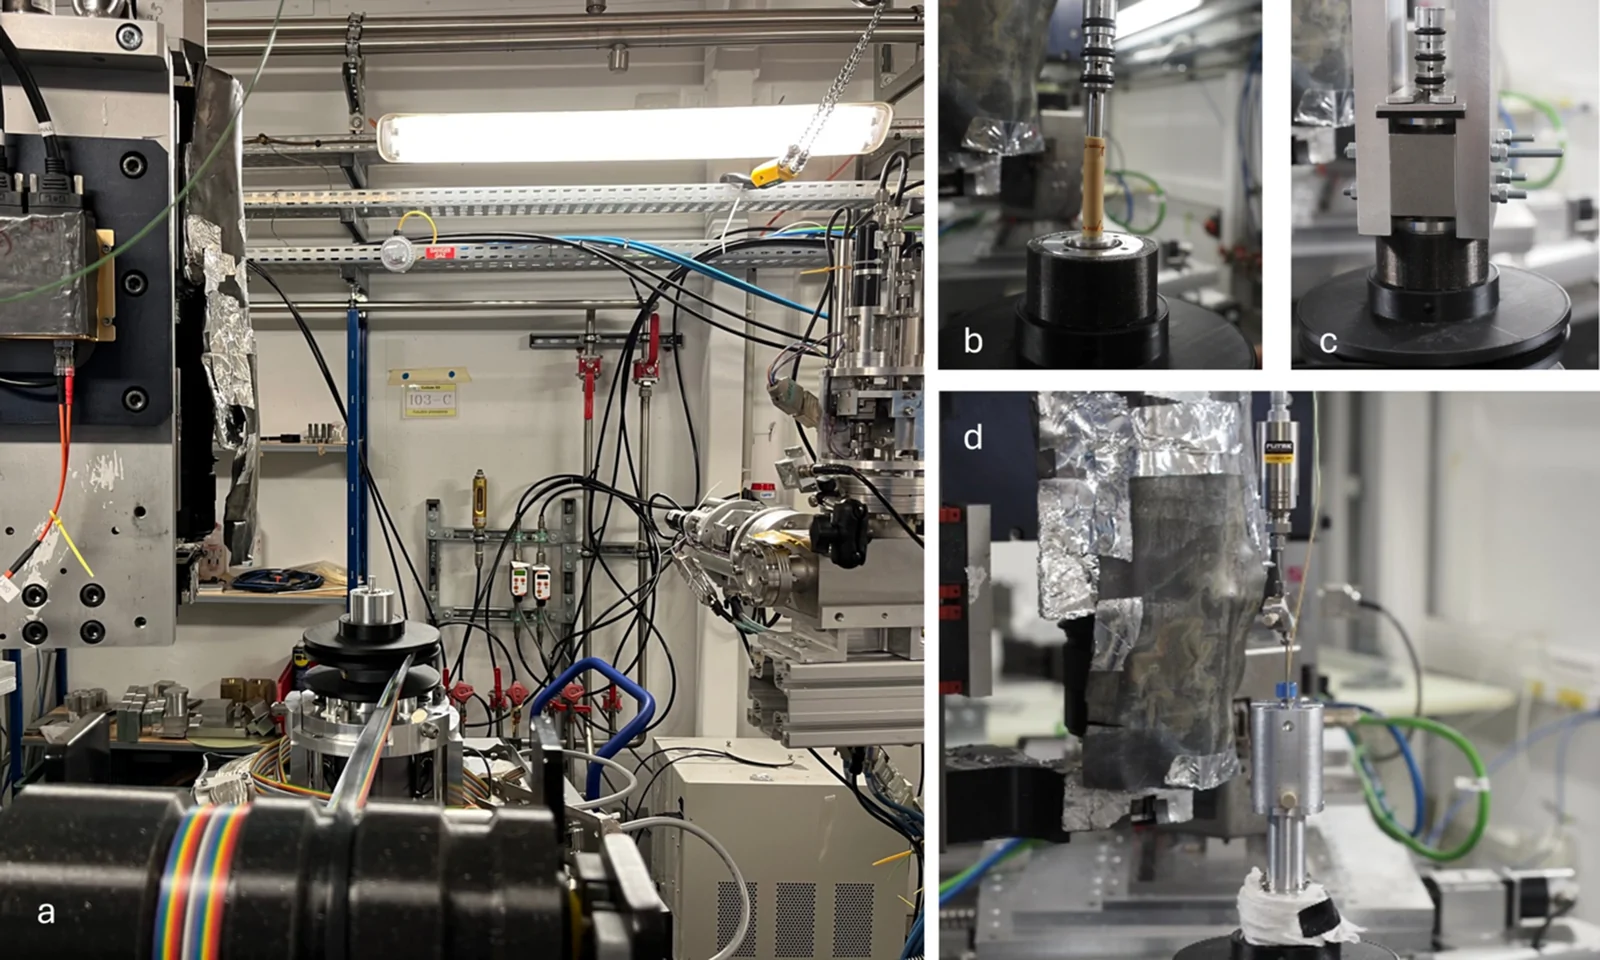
(*a*) Triaxial press base set in the optical hutch of the PSICHE beamline (Synchrotron SOLEIL) on the X-ray beam path, with the detector on the left. (*b*) 8 mm sample in its jacket, with copper wires tightening the silicone jacket against the pistons. (*c*) Mounting aid allowing to carefully screw the top piston inside the head and control the force applied on the sample. (*d*) Final setup, with the PEEK tube and the confining pressure sensor attached to the head.
